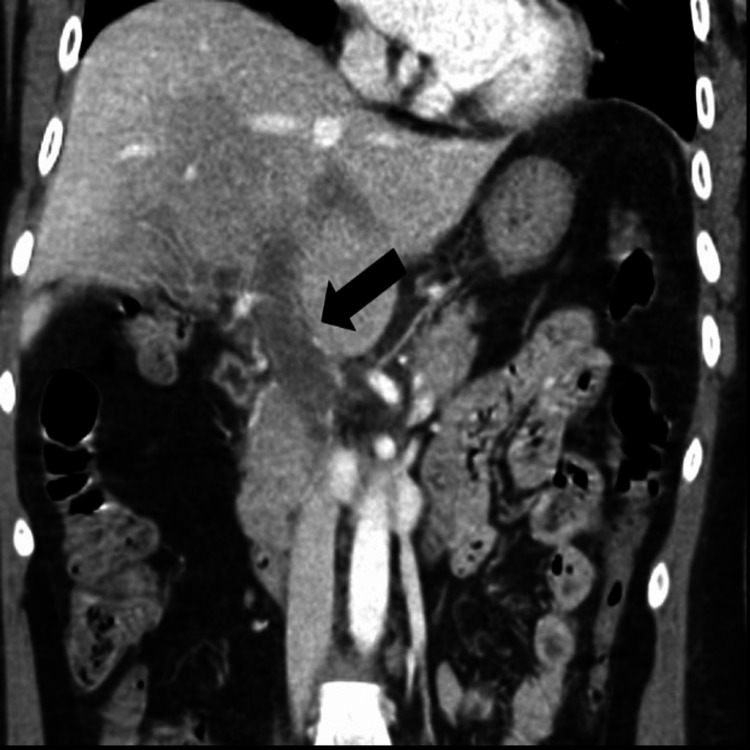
CT abdomen and pelvis, coronal image, shows a large thrombus in the main portal vein (arrow) extending into the right and left portal venous branches.
